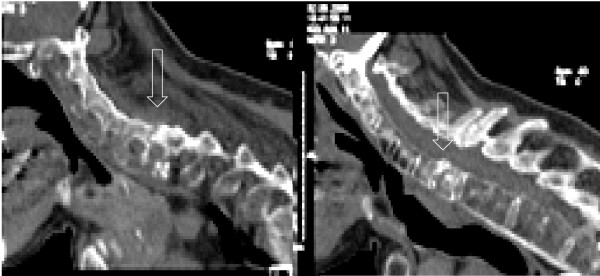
The CT 2-D reconstruction shows a thin fracture line in a completely but not dislocated fracture at C6/7.
